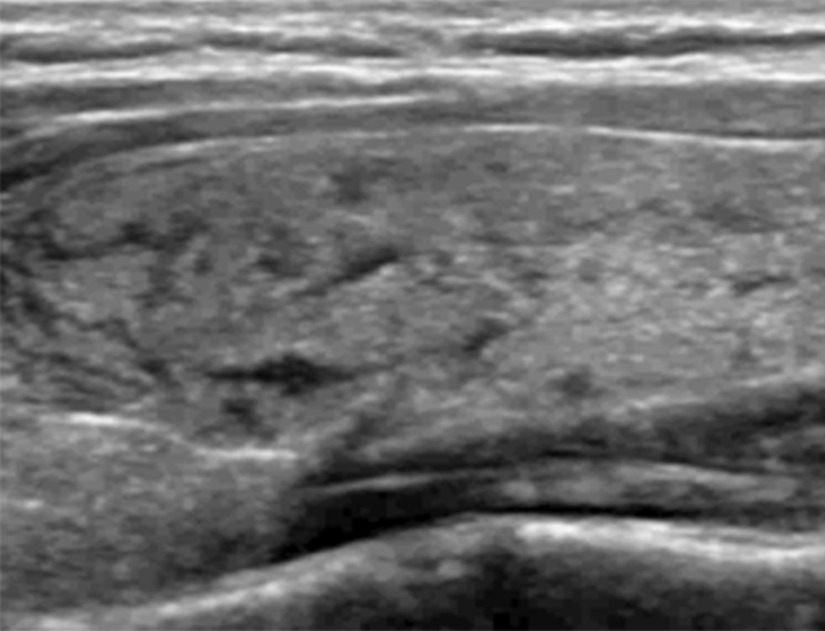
Ultrasonography showed the left thyroid lobe was swelling with multiple funicular hypoechoic lesions
